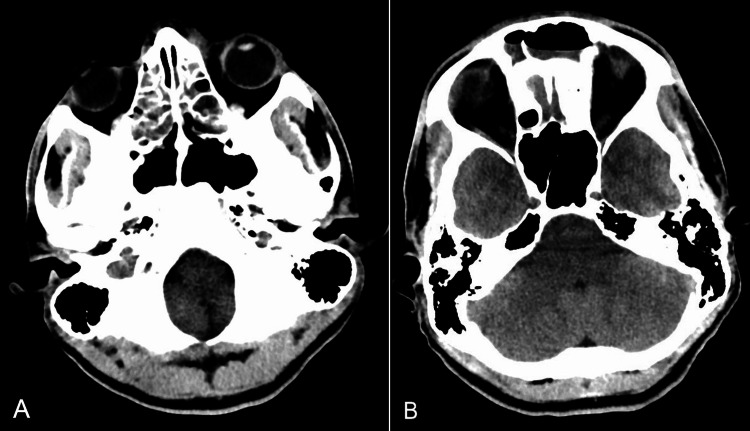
Head CT scan showing marked opacification of paranasal sinuses (A) with air-fluid level in the frontal sinuses (B). CT: computed tomography
